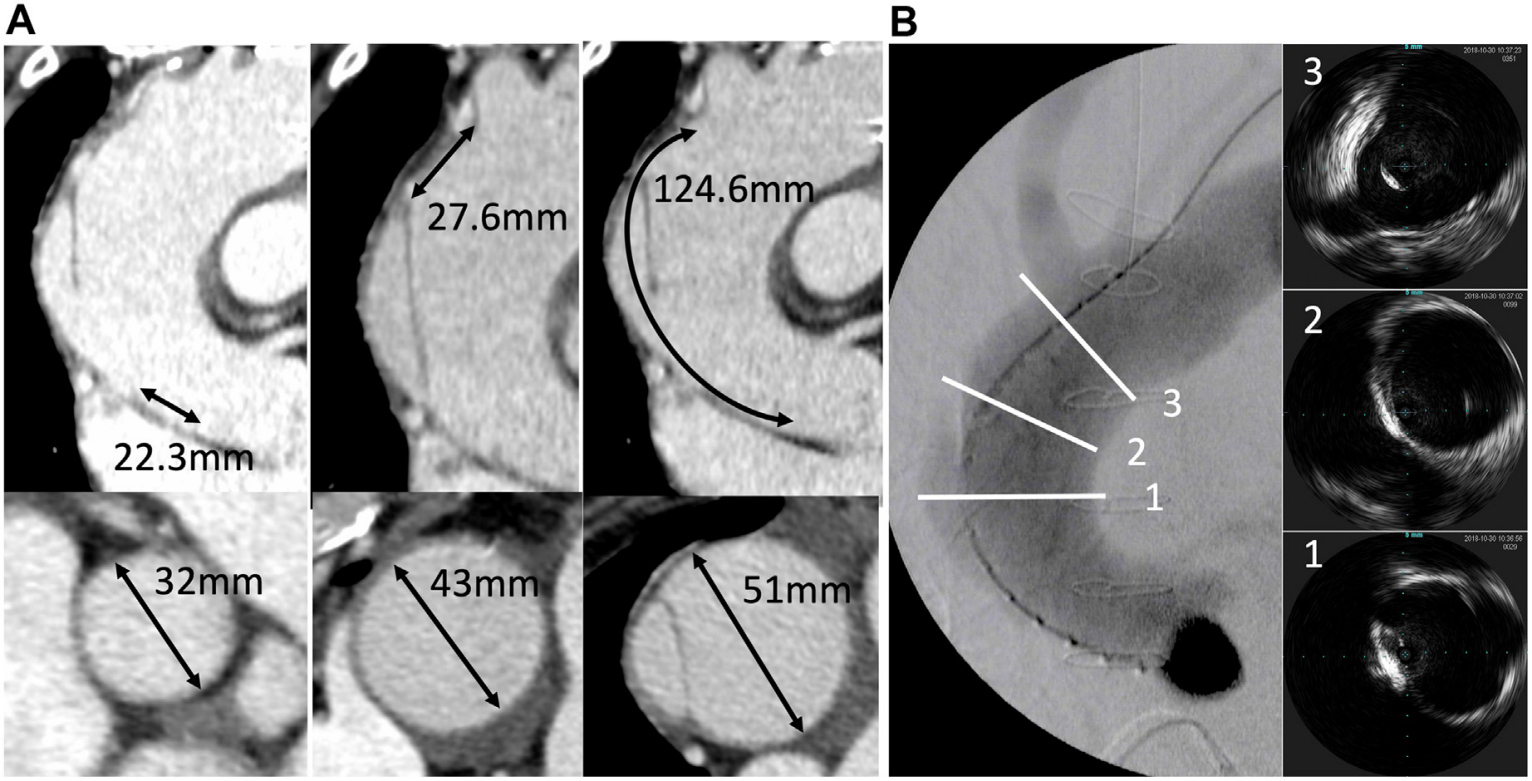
(A) Contrast-enhanced computed tomography scan before thoracic endovascular aortic repair. The proximal diameter and landing zone were 32 mm and 22.3 mm; the distal diameter and landing zone were 43 mm and 27.6 mm; the maximum diameter and length of the greater curvature of the ascending aorta were 51 mm and 124.6 mm, respectively. (B) Intraoperative intravascular ultrasound image (during thoracic endovascular aortic repair) showing (1) an open false lumen in the ascending aorta, (2) distal rounding zone, and (3) brachiocephalic trunk. Length of the ascending aorta >100 mm.
